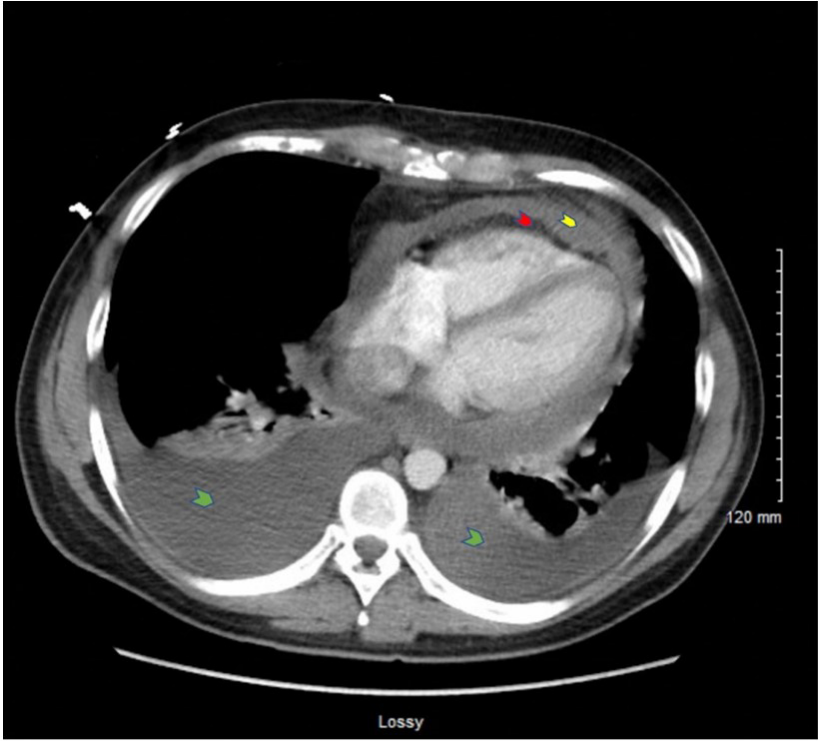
CT chest angiogram demonstrating moderate to large-sized bilateral pleural effusions, greater on the right side (green arrowheads), moderate-sized pericardial effusion (red arrowhead), thickened pericardium (yellow arrowhead), along with mediastinal and hilar adenopathy, and mediastinal fat stranding.
